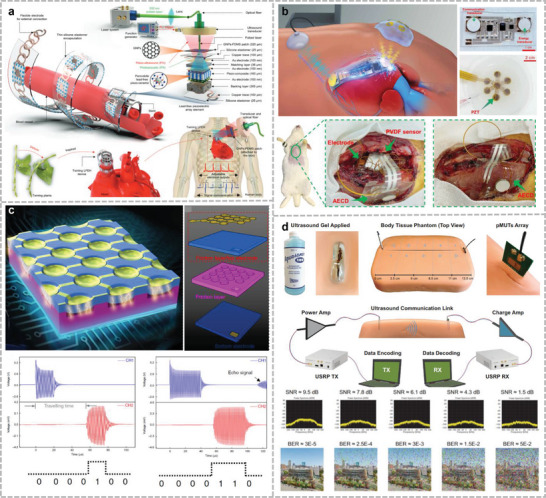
Ultrasonic transducer for in vivo communication. a) A novel design and implementation of a hybrid induced energy transfer strategy using photoacoustic (PA) and piezo‐ultrasound (PU) technology in a 3D twining wireless implant. Reproduced with permission.^[^
